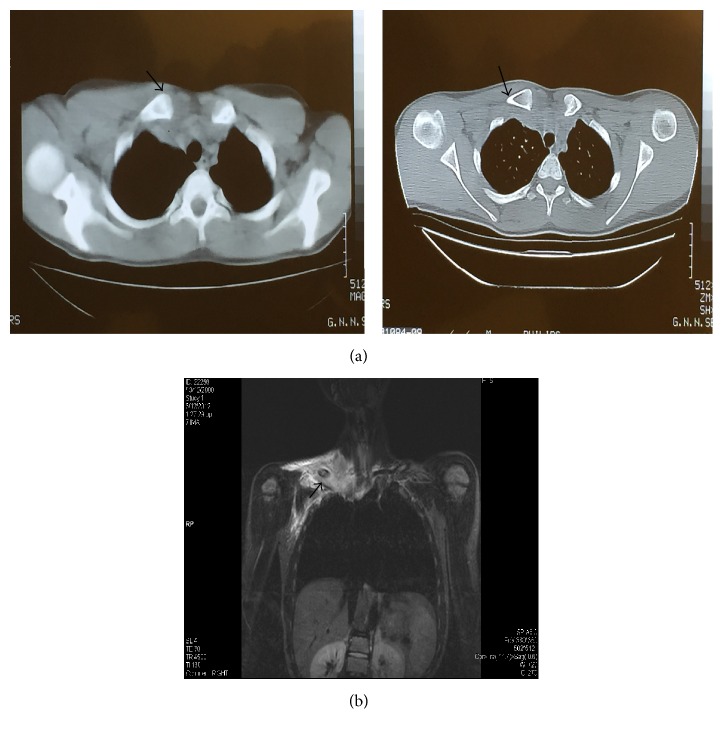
(a) The CT scan showed widening of the soft tissues and the proximal part of the right clavicle without obvious change in the bone marrow and without erosion of the cortex. (b) The MRI showed abnormal signal in the right clavicle with periosteal reaction.
